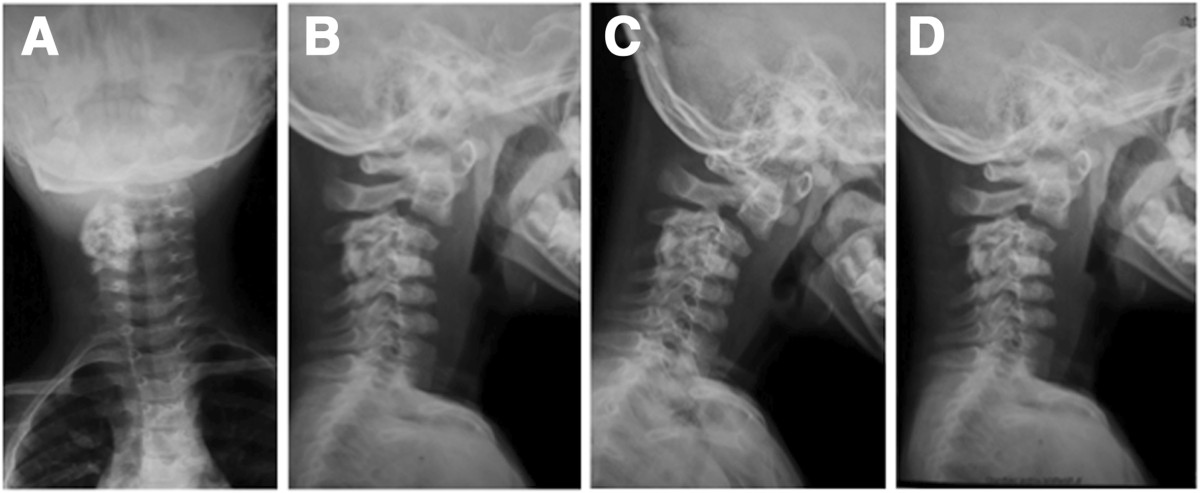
Preoperative radiographs. (A): Anteroposterior view shows an oval high-intensity area and a well-defined rim from C3 to C4 in the right side. (B): Lateral radiograph shows a lesion arising from the posterior arch of C3-4. (C) and (D): Postoperative anteroposterior and lateral plain radiographs show the tumor has been removed grossly and internal fixation has been performed.
